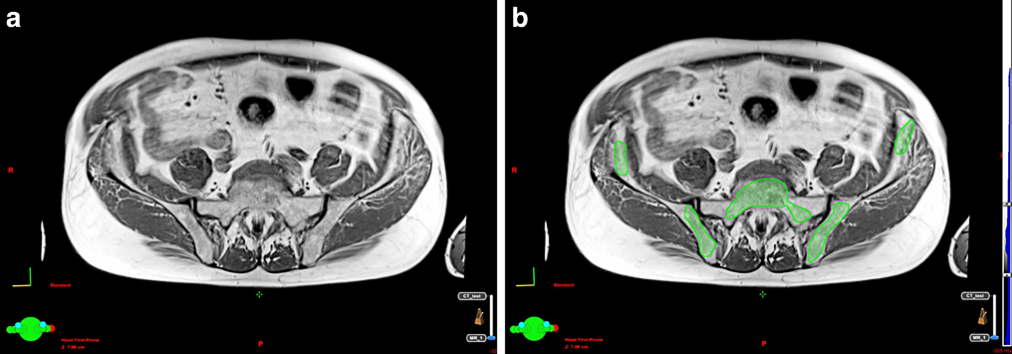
Contouring method of active bone marrow based on magnetic resonance imaging. a T1-weighted transverse MRI image, b T1-weighted transverse image with active bone marrow contour
